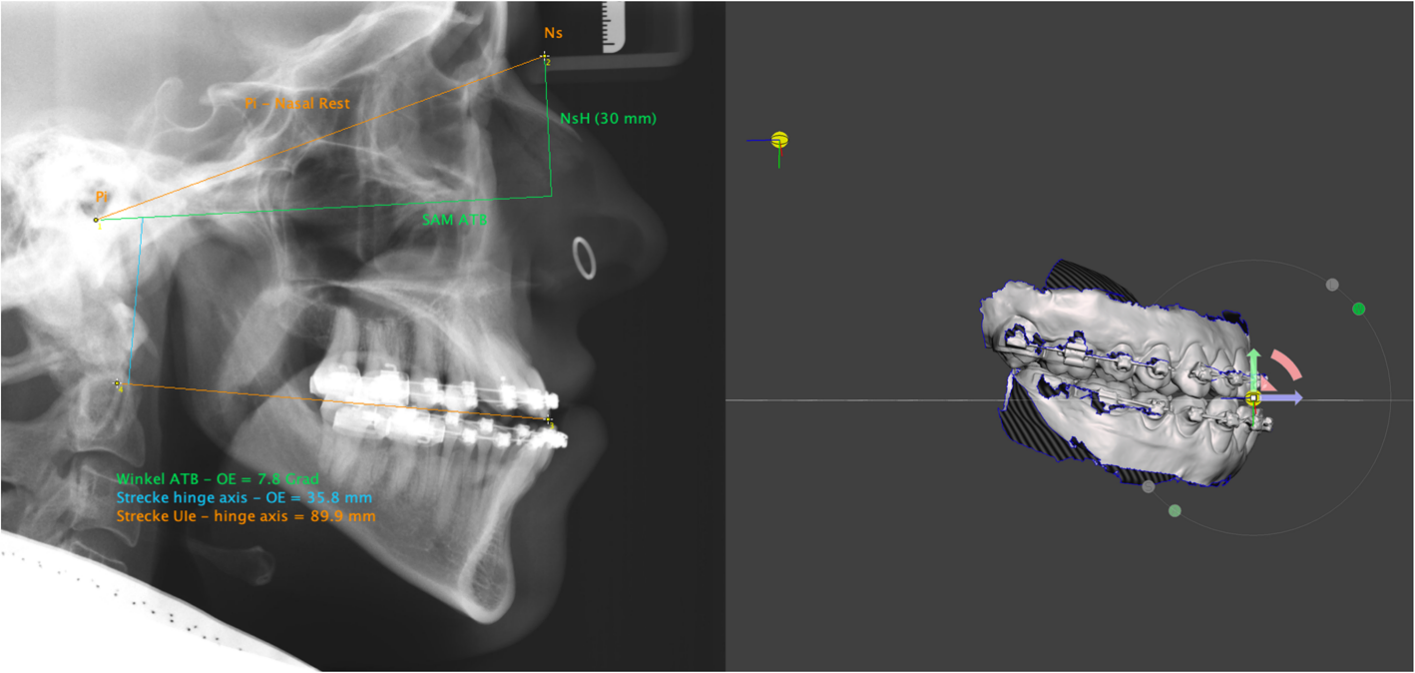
Left: The following calculations are projected on the cephalometric x-ray: Angle between upper occlusal plane and AOP (7.8^∘^), distance from the articulator’s hinge axis perpendicular to the upper occlusal plane (35.8*mm*), and the distance from the latter intersection to the upper incisor edge (89.9*mm*). Right: Movement of the hinge axis pivot according to the calculated distances following clock wise rotation of the all objects around upper incisor edge. Upper and lower jaw are thereby arbitrarily aligned to the AOP in Meshmixer’s world frame
